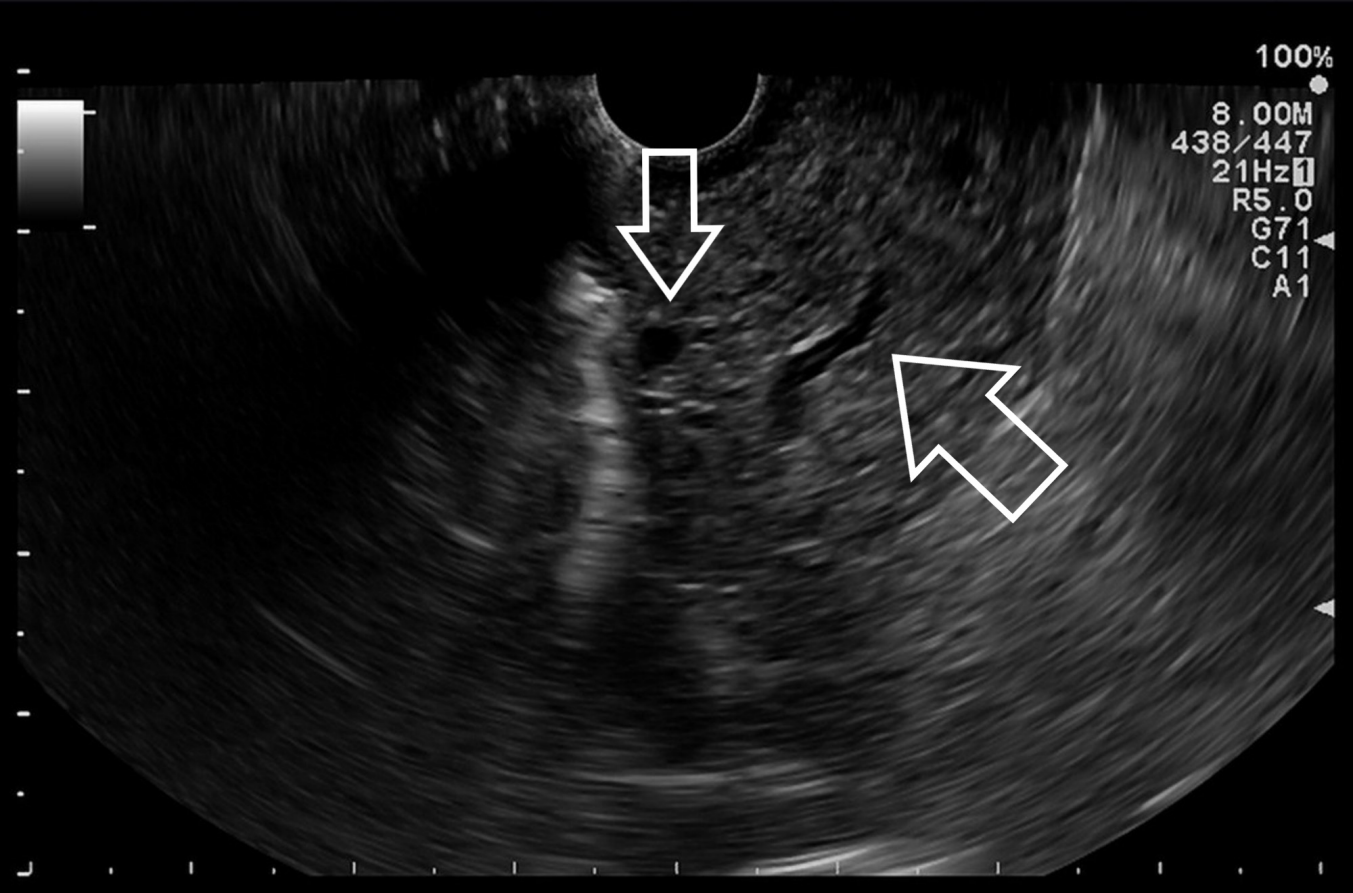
Endoscopic ultrasonography. Mixed echoic mass without a clear distal margin in the fourth layer of the gastric wall; some ductal images are evident (arrows).
